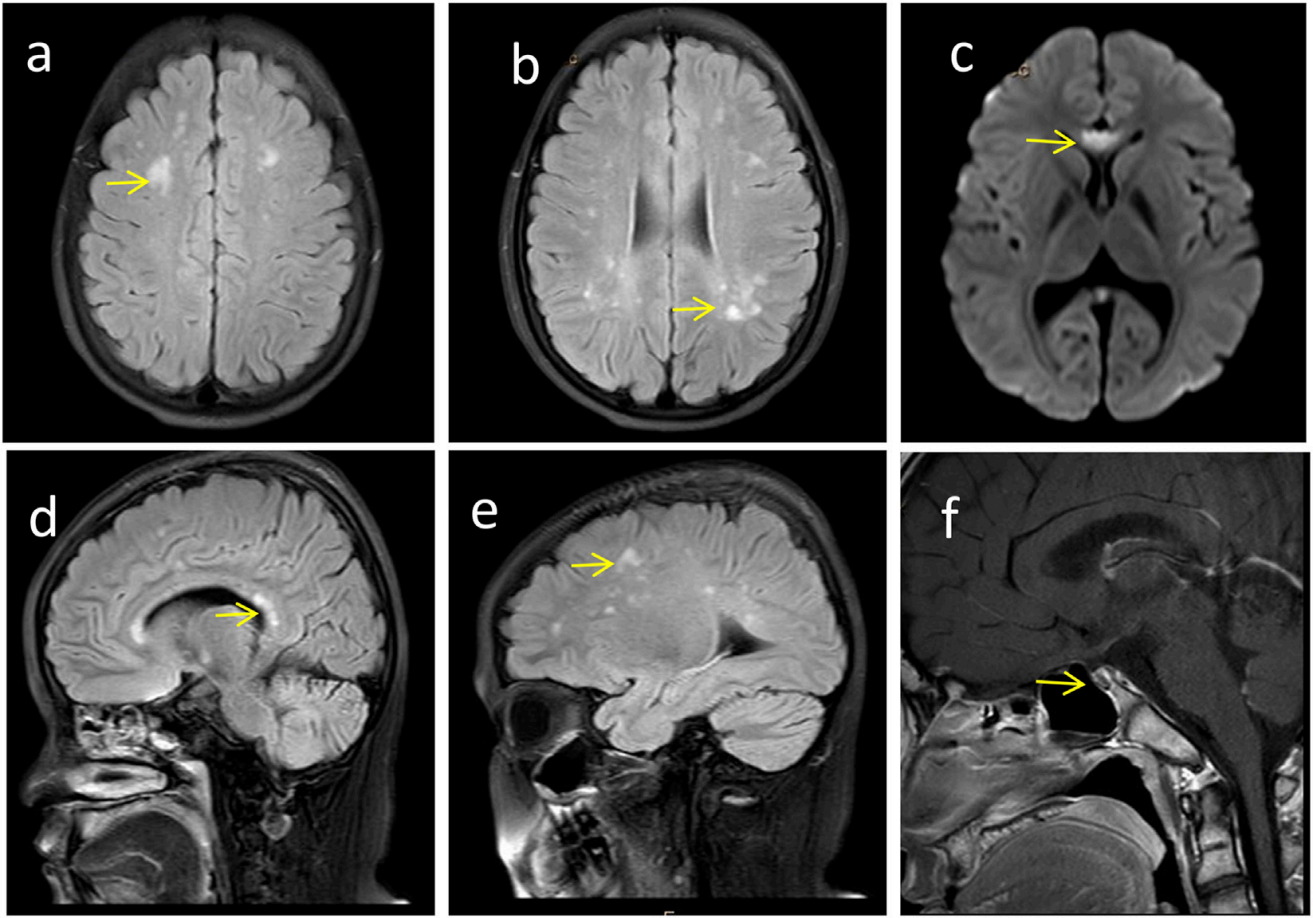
Craniocerebral imaging findings of the proband. (a-e) Brain MRI reveals high-signal white matter lesions (→ indicated), which involve the bilateral centrum semiovale, periventricular regions, as well as the genu and splenium of the corpus callosum. (f) Enhanced MRI of the pituitary gland demonstrates pituitary atrophy (→ indicated).
